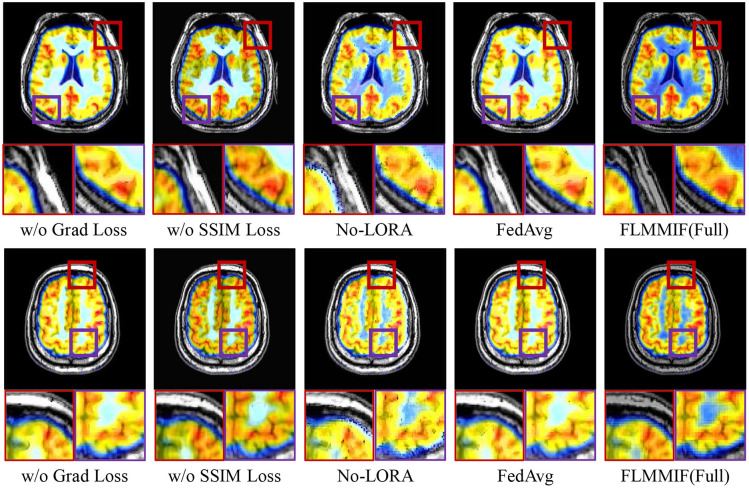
Quantitative assessment of ablation study on PET-MRI dataset.
